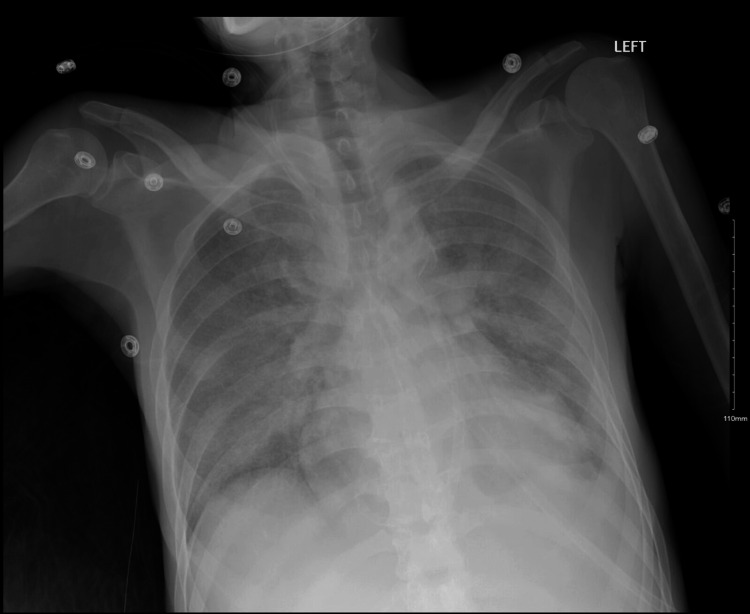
Chest x-ray showing diffuse ground-glass consolidation concerning for pneumonia or edema.
